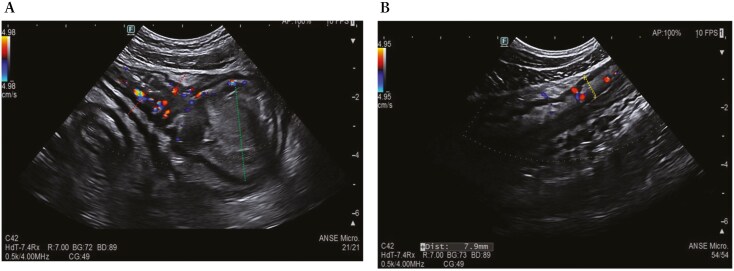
Intestinal ultrasound shows thickening of the terminal ileum (red dotted line) with increased vascularization and upstream dilation (green dotted line) (A) and parietal thickening of the sigmoid colon up to 7.9 mm (yellow dotted line) with increased vascularization in the submucosa and narrowing of the lumen (B).
